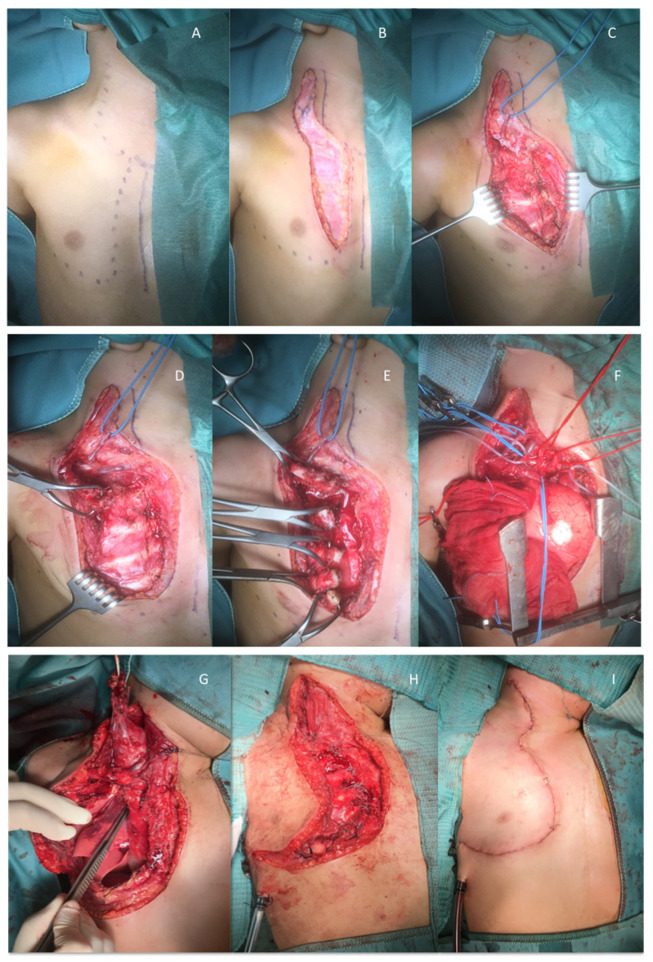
Main steps of the Cervico-Parasternal Thoracotomy (CPT). (A). Incision line for CPT type A. (B,C). Surgical dissection of the subcutaneous tissue. (D,E). Detachment of the major pectoralis muscle and identification and disarticulation of the clavicle and the ribs from their sternal insertion. (F). Identification and isolation of vital structures: common carotid artery (red elastic wire), subclavian artery (red elastic wire), internal jugular vein (blue elastic wire), phrenic nerve (white elastic wire). (G–I). Main steps and incision line for CPT type B.
